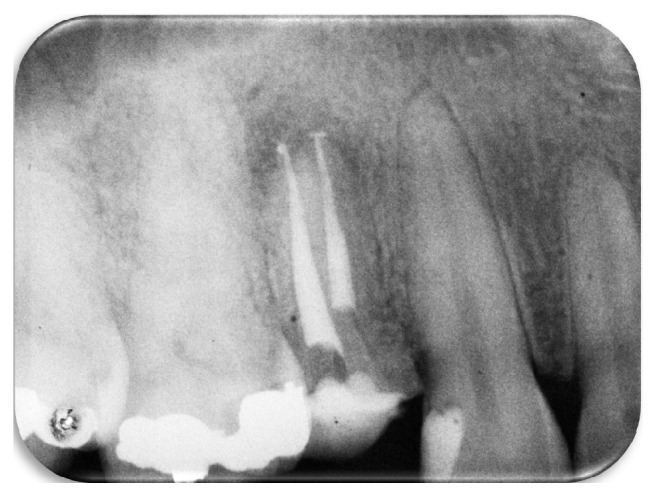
Periapical radiograph of the endodontic treatment completed.
